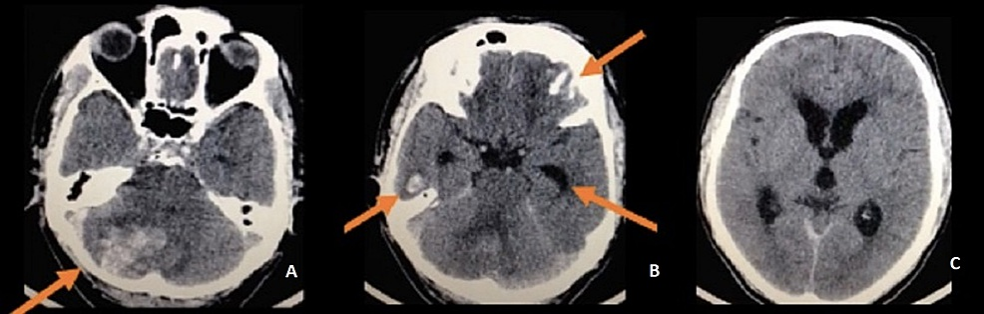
Initial non-contrast computed tomography (CT) scan (A) Axial view orange arrow showing cerebellar traumatic hematoma with a narrow fourth ventricle and lateral displacement. (B) Orange arrows showing wide temporal horns, temporal and frontal countercoup contusions. (C) Non-contrast CT scan with signs of hydrocephalus.
